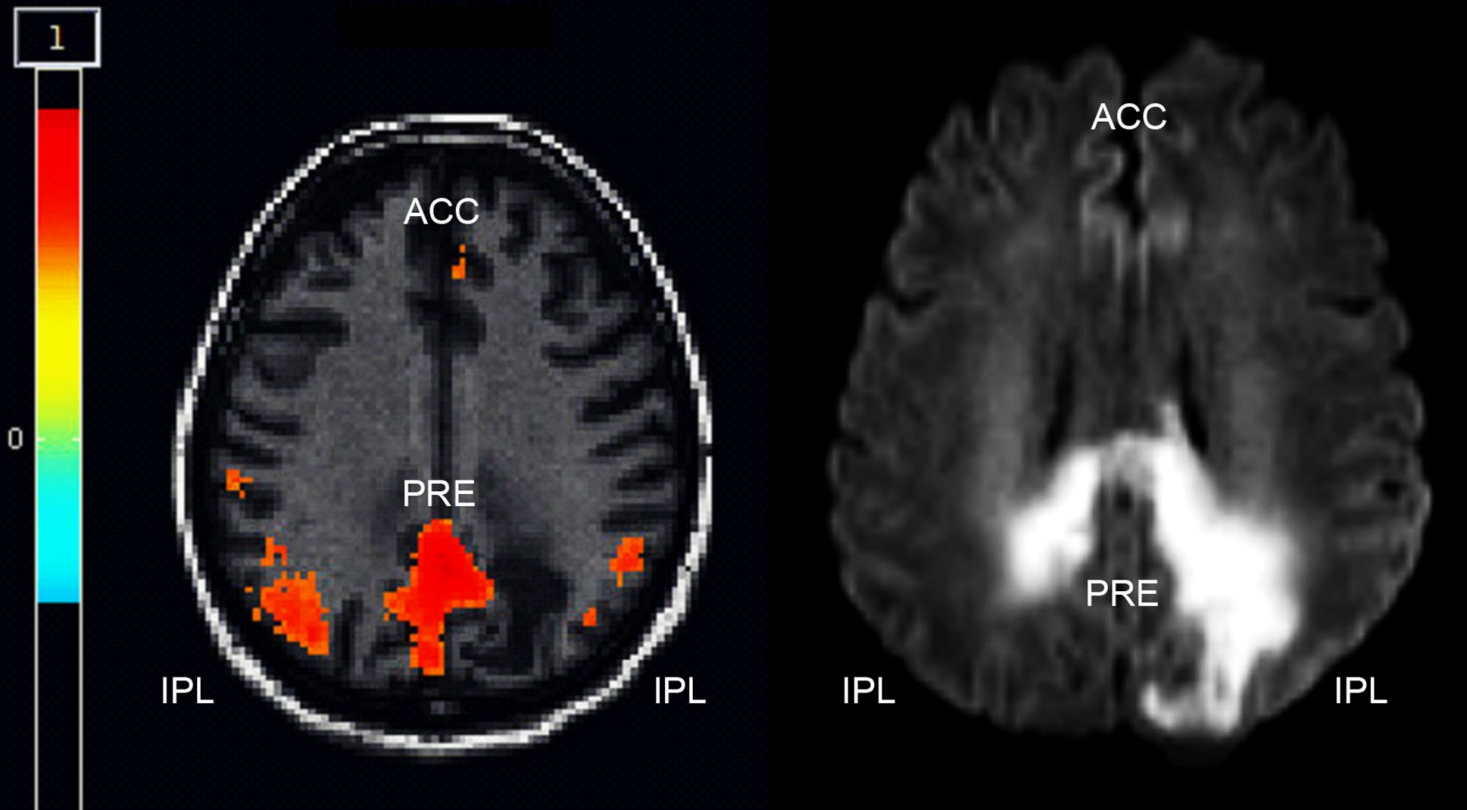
Resting state functional MRI (with central seed placed in the precuneus, PRE). *IPL* and *ACC* denote inferior parietal lobe and anterior cingulate cortex, respectively. Images in neurological convention; *red areas* indicate connectivity between PRE and IPC/ACC >0.5 (Whitfield-Gabrieli and Nieto-Castanon 2012). *Left* multiband EPI-sequence (TR 300 ms, TE 30 ms, 32 contiguous slices, matrix size 64 × 64, FoV 1380 mm); *right* diffusion weighted imaging sequence (b = 1000, TE 3500 ms, TE 89 ms, matrix size 128 × 128, FoV 230 mm)
